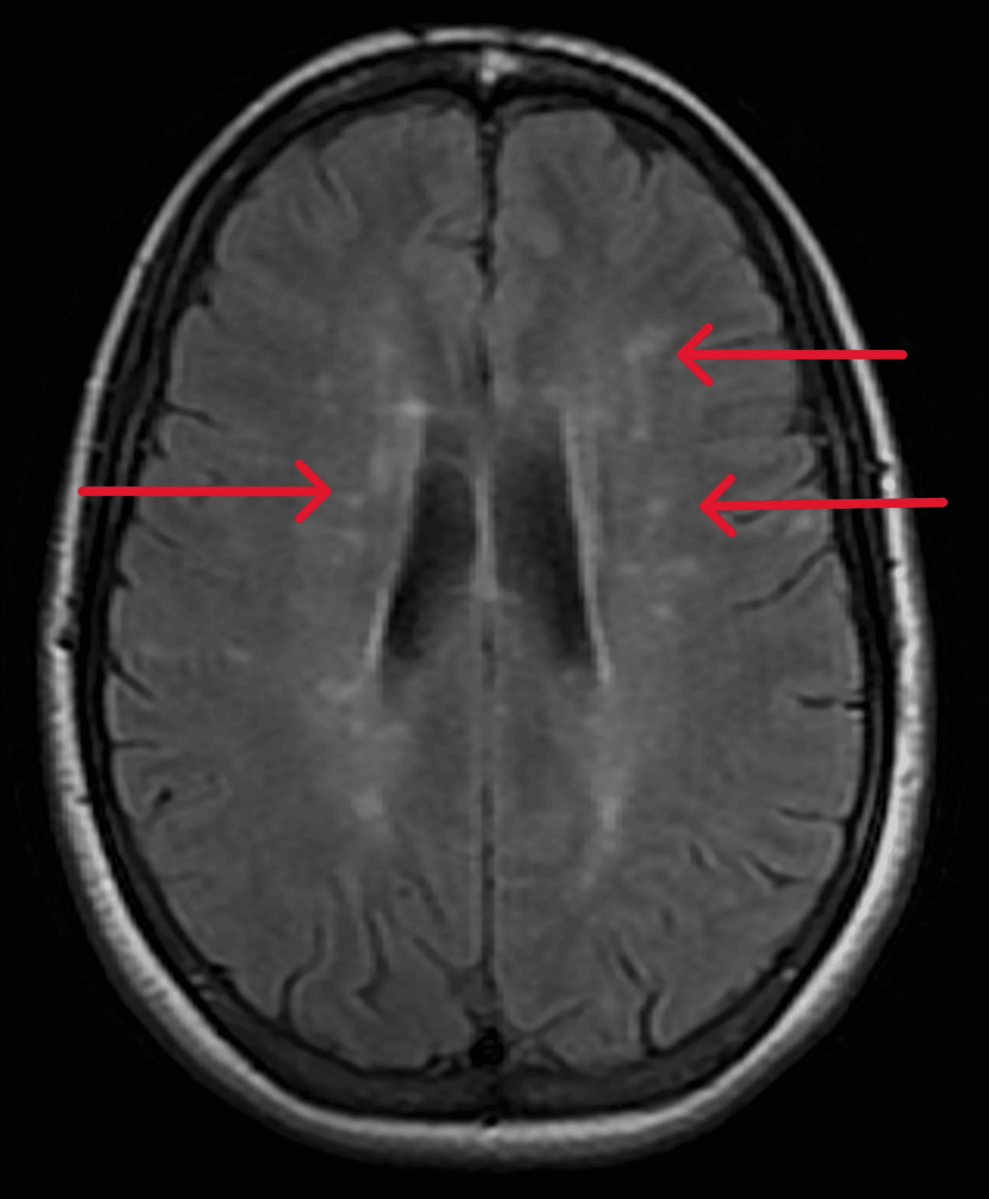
Axial T2-FLAIR MRI demonstrating hyperintense white matter lesions characteristic of multiple sclerosis, stable compared to the previous MRI. The arrows highlight the periventricular lesions of multiple sclerosis (MS). FLAIR: fluid-attenuated inversion recovery
